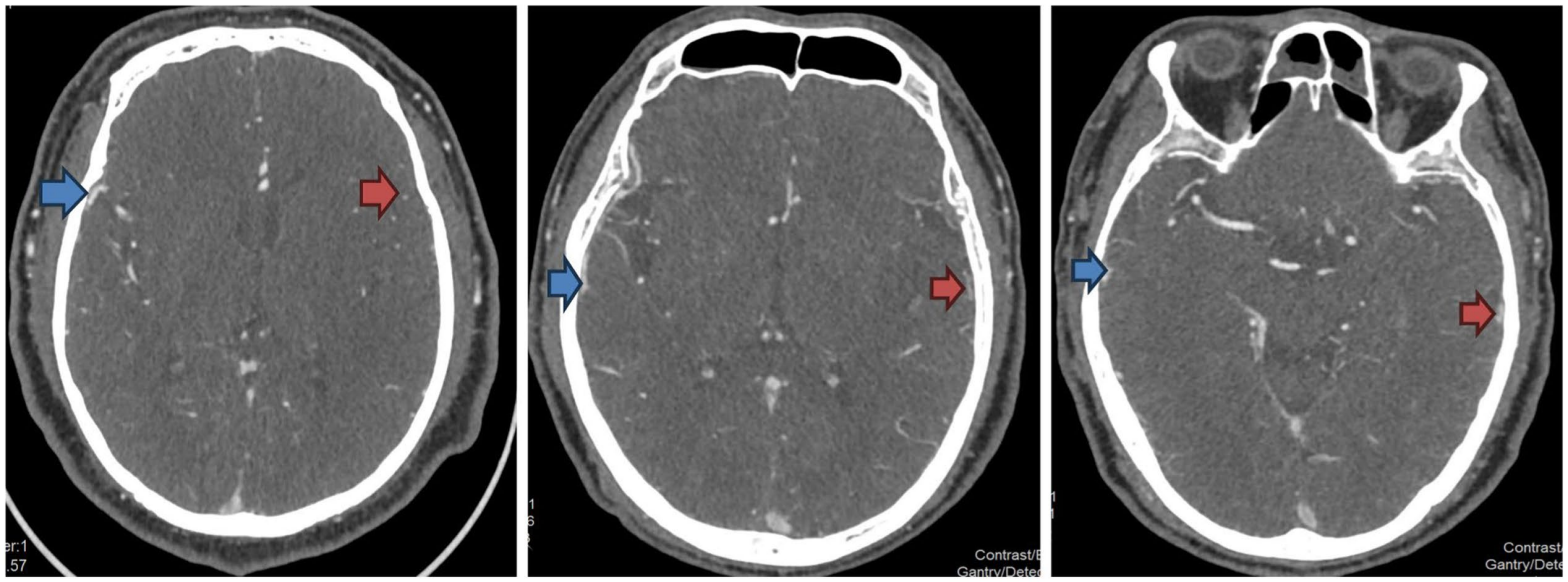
Examples of the CTA vein opacification score. The side indicated by the red arrow is the affected side, and the side indicated by the blue arrow is the healthy side. From left to right in the figure, the venous opacities are scored from 0 to 2 points, respectively.
